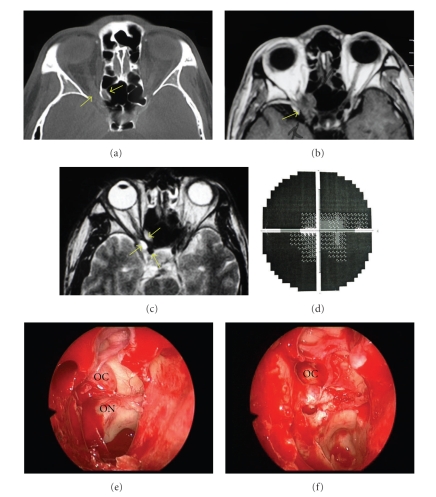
(a) Orbital CT imaging of a right Onodi cell mucocele compressing the optic nerve (arrows). (b) T1-weighted MRI image showing a hypointense signal due to the water content in the Onodi cell (arrows). (c) T2-weighted MRI image with a high signal intensity within the Onodi cell (arrows). (d) Humphrey visual field of the right eye with significant constriction of the visual field. (e) A clinical photograph of the Onodi cell mucocele (OC) and the optic nerve (ON) prior to decompression. (f) A clinical photograph following marsupialisation of the Onodi cell and drainage of the mucocele (OC).
